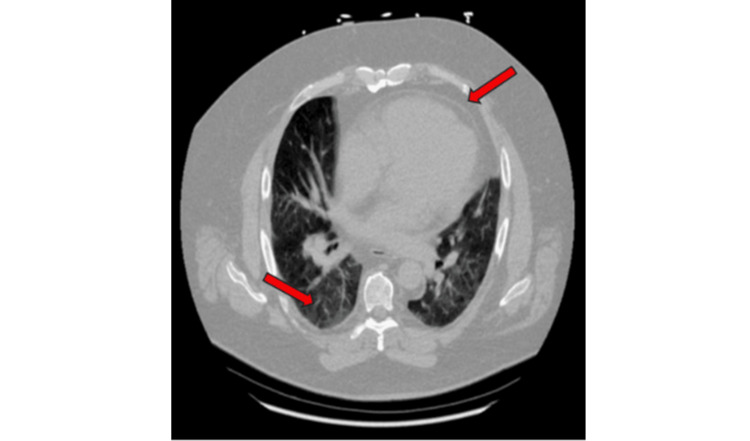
Chest computed tomography of the lung Chest computed tomography showing ground-glass opacities of the right lung and mild pericardial effusion.
